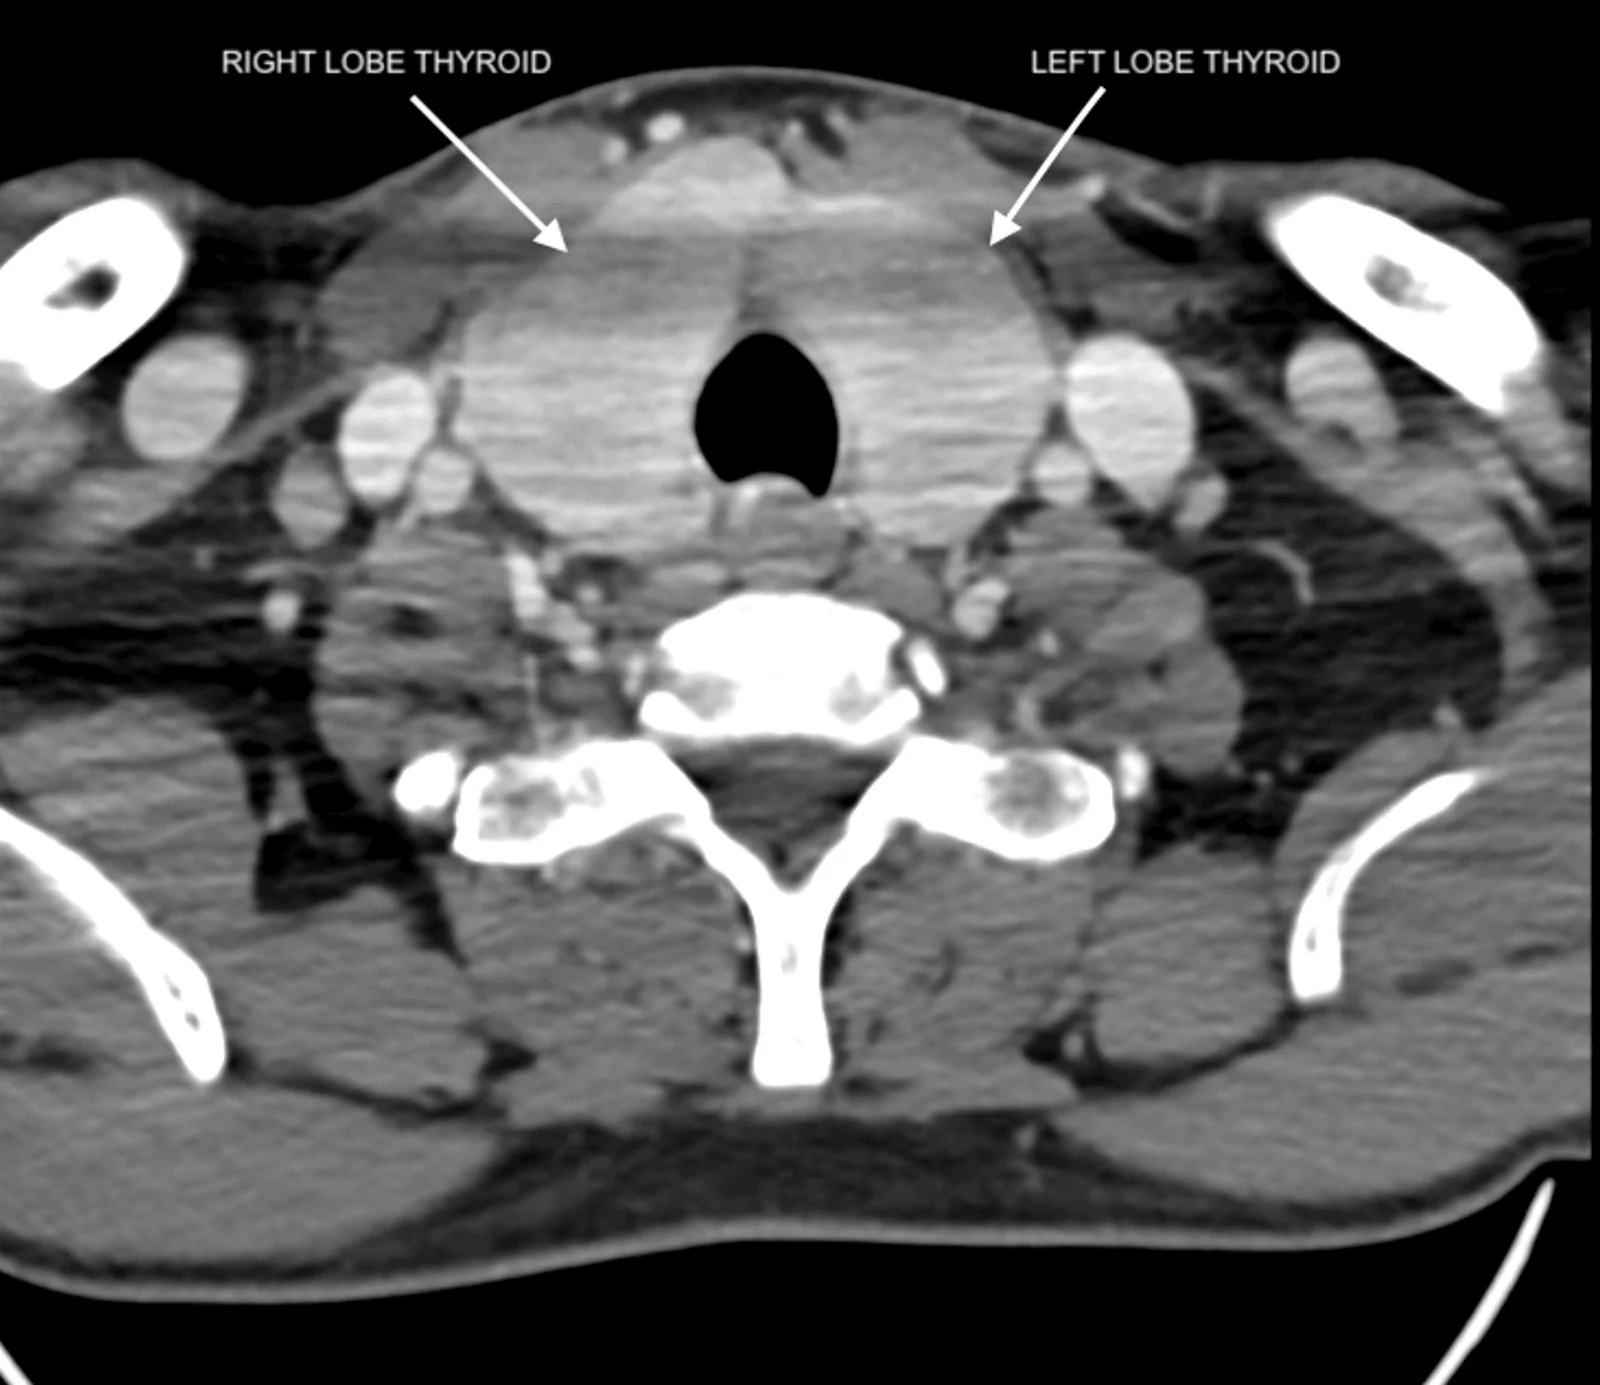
Contrast-enhanced axial CT of the chest with thyroid dimensions of 7.6 × 5.2 cm in transverse diameter and 7.9 cm craniocaudally.
